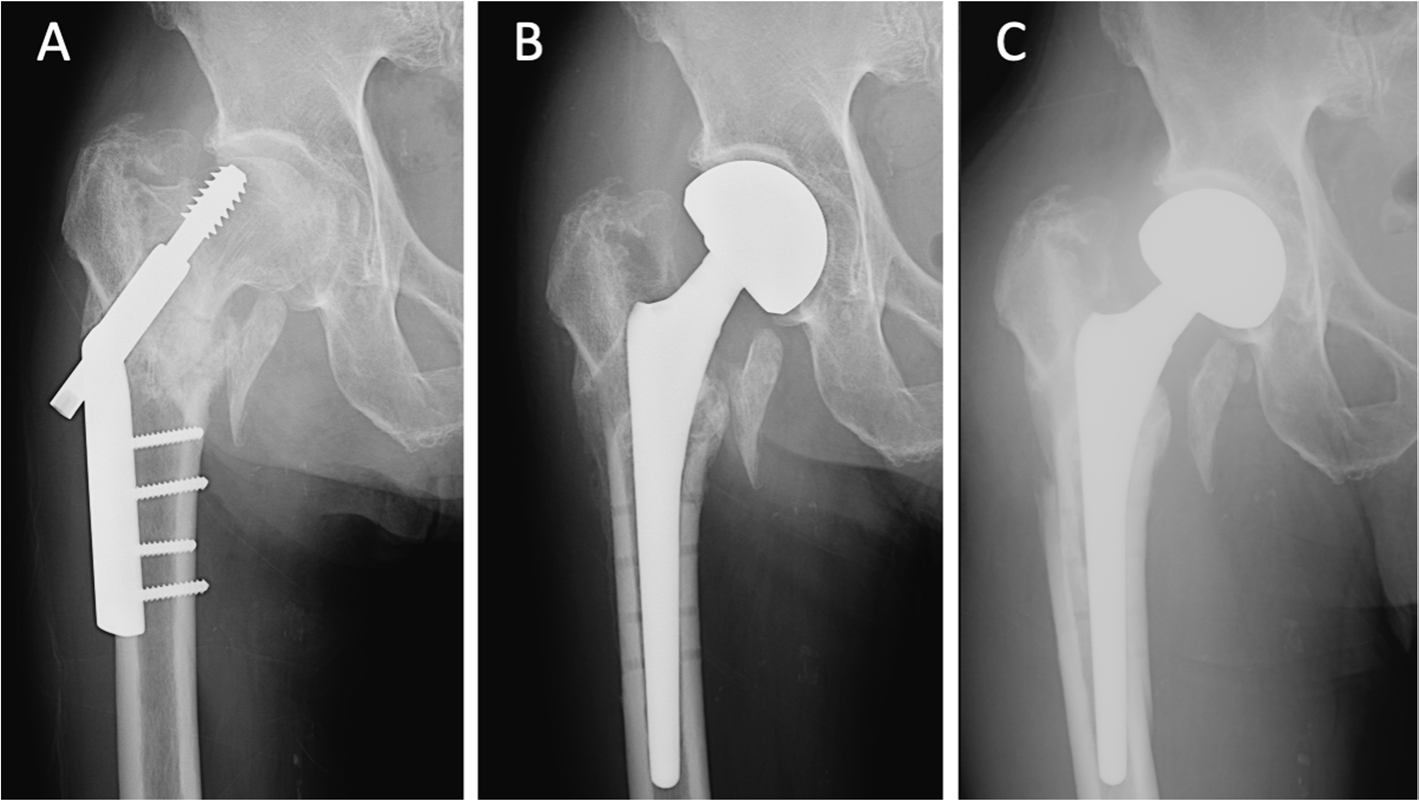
(A) 82-year-old male, with early fixation failure (postoperative 6 weeks, fracture collapse), (B) bipolar hemiarthroplasty with cementless primary stem, immediate postoperative radiograph, (C) periprosthetic femoral fracture at postoperative 4 months
